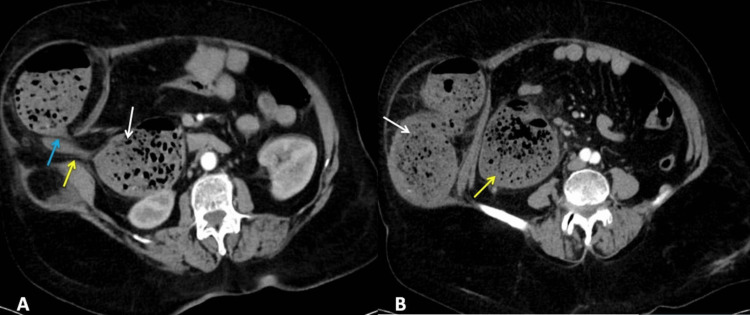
Computed tomography (axial view) showing (A) previous drain site (yellow arrow), grossly dilated right colon (white arrow), volvulus of the herniated transverse colon (blue arrow), (B) a grossly dilated segment of the transverse colon (white arrow) and grossly dilated right colon (yellow arrow).
